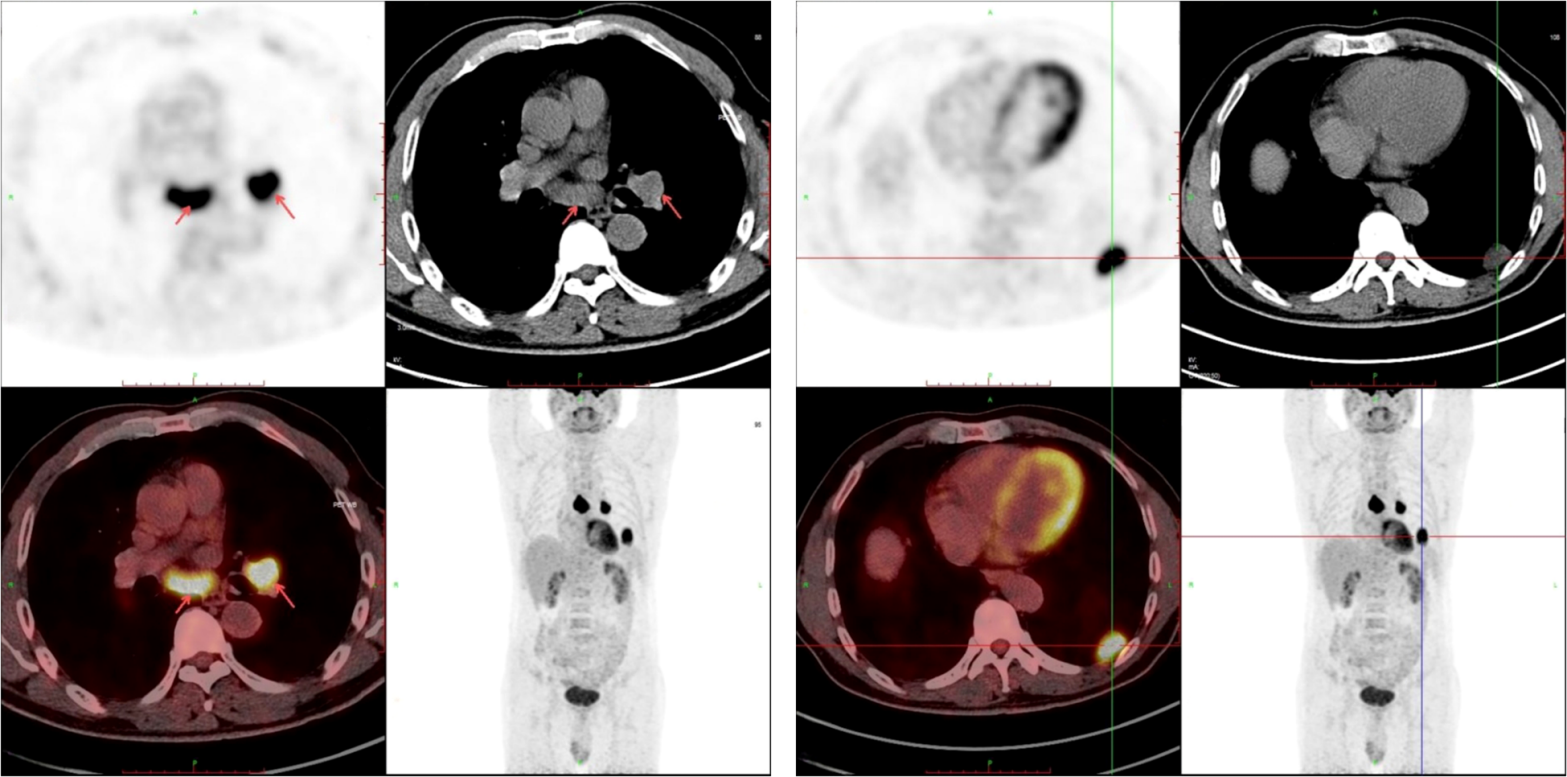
The PET-CT scan of this patient indicated possible left lower lobe malignancy with mediastinal and hilar lymph node metastases.
